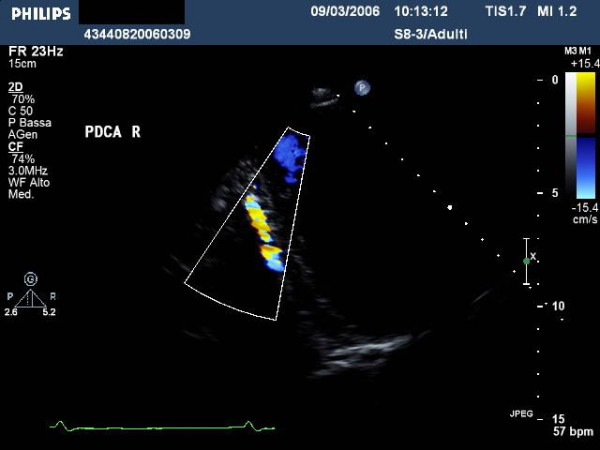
highlights the Ultrasound findings of distal left posterior descending coronary artery highlighted with Color-Doppler.
